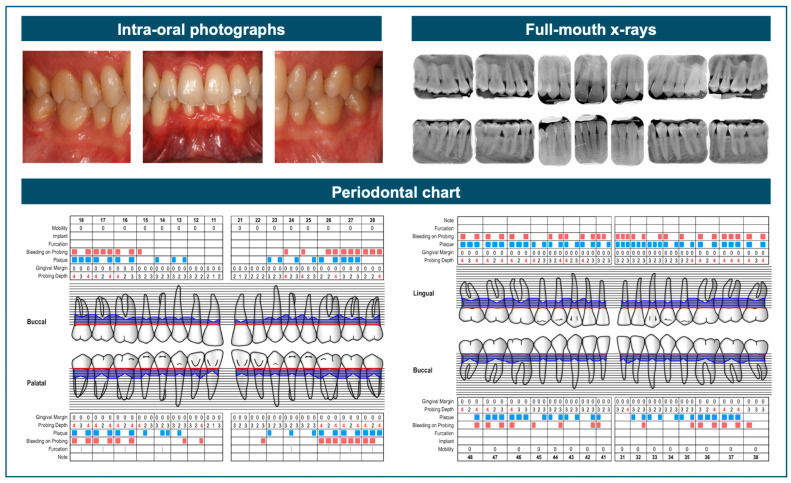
This case falls within a diagnostic gray zone due to the presence of gingival inflammation, characterized by redness, edema, and bleeding on probing, along with sites exhibiting probing depths of 4 mm. Radiographically, there is no evident bone loss reaching one-third of the root length, and only minor qualitative changes in the bone structure can be observed. It is important to distinguish true periodontal pockets from potential pseudo-pockets in order to accurately assess the presence of clinical attachment loss, which in this case would be minimal.
